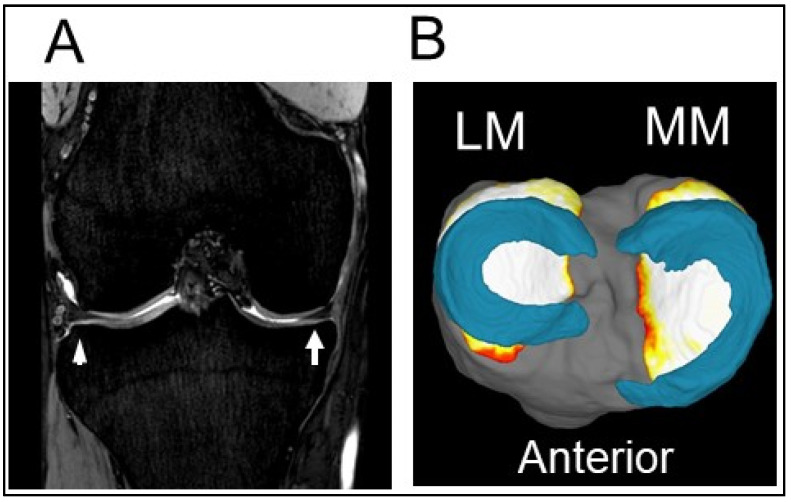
A normal meniscus in the right knee. (A) Coronal view of the normal meniscus. Arrow indicates the MM. Arrowhead indicates the LM. (B) A 3D MRI view reconstructed using SYNAPSE 3D software (Japanese product name: SYNAPSE VINCENT; FUJIFILM Corporation, Tokyo, Japan). MM, medial meniscus; LM, lateral meniscus; MTP, medial tibial plateau.
